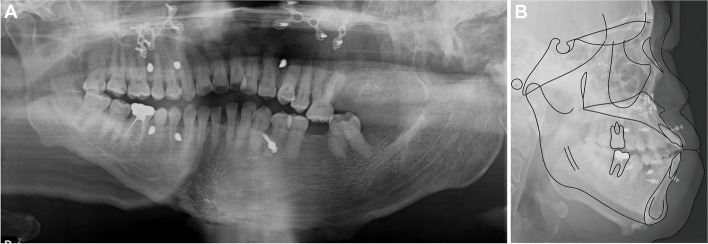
One-month post-arthroplasty radiographs. A Panoramic radiographs, B Lateral cephalogram and tracing
